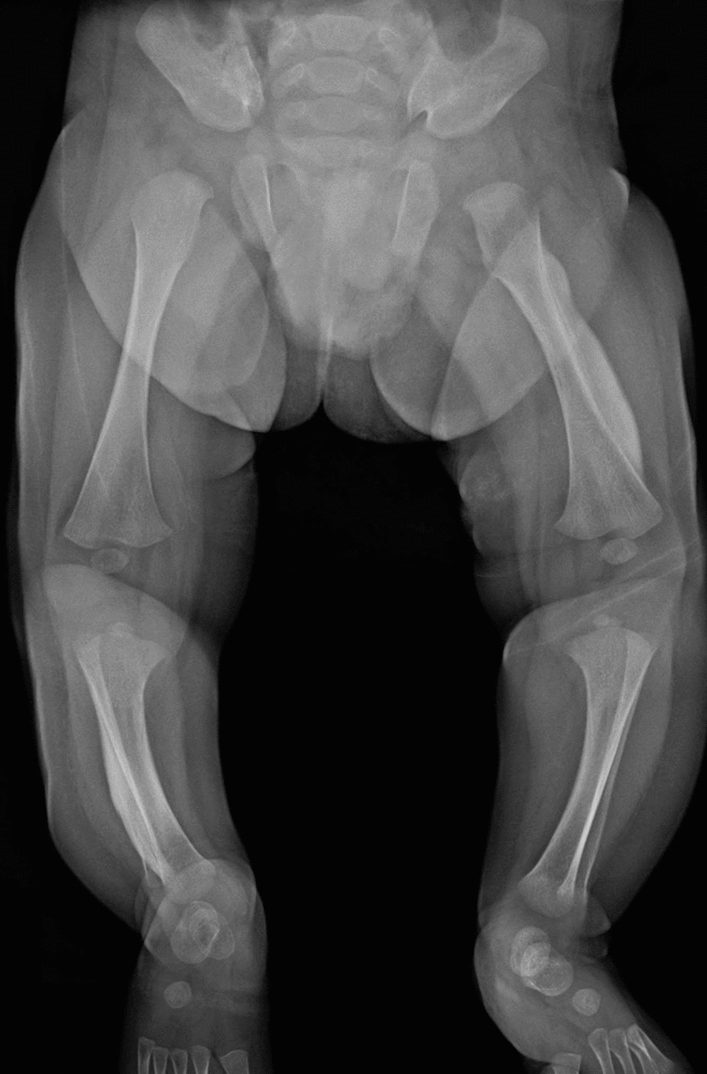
A 2-month-old infant with infantile cortical hyperostosis (Caffey disease). Radiograph shows cortical hyperostosis in the left femur and right tibia
